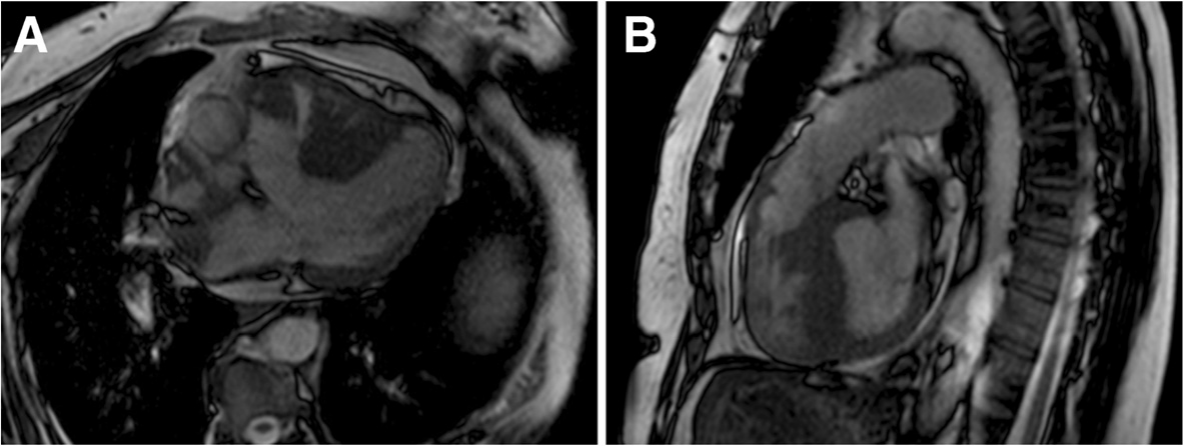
Cardiac magnetic resonance imaging confirming the diagnosis of tetralogy of Fallot. A: Ventricular septal defect and overriding aorta over the septum. B: Right ventricular hypertrophy and subpulmonary stenosis.
